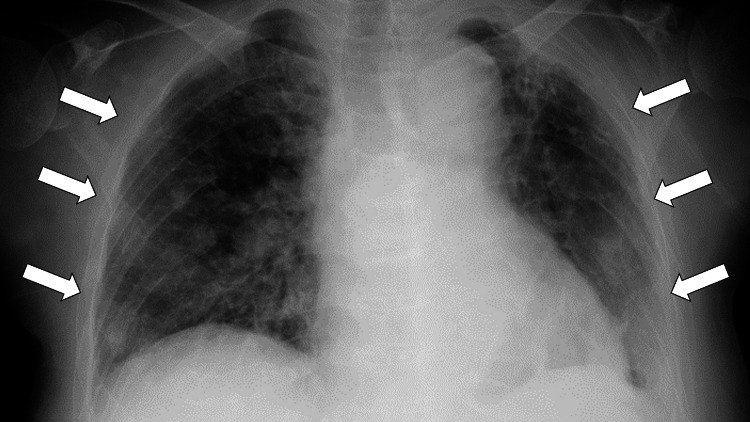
A chest X-ray demonstrating bilateral pulmonary congestion and pleural effusion
